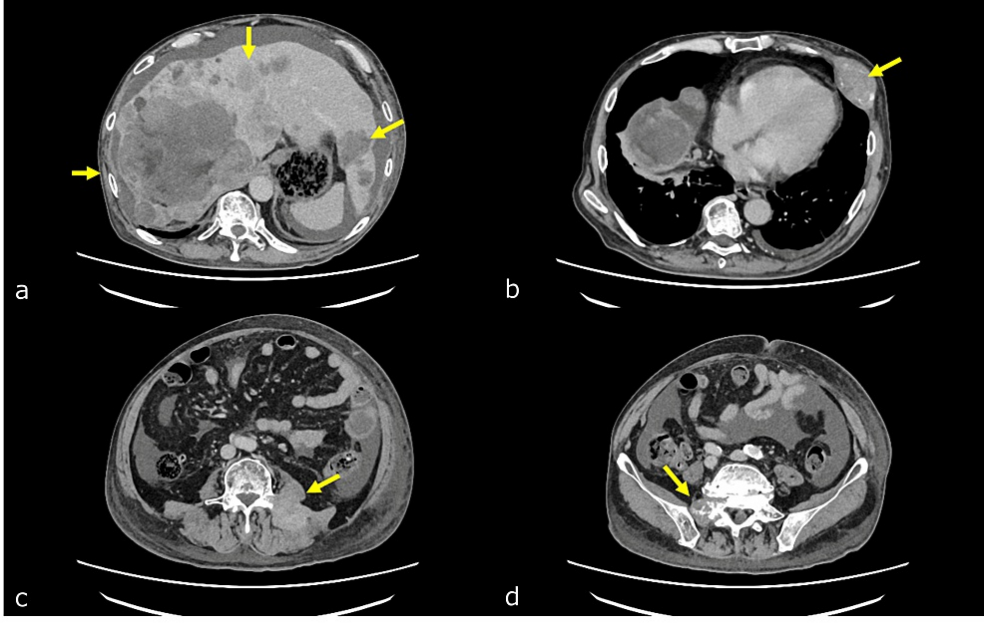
CT scan of the trunk A CT scan of the trunk, lumbar region, and pelvis revealed advanced liver metastases (a, indicated by yellow arrow) and metastatic tumors in the rib (b, indicated by yellow arrow), sacrum (c, indicated by yellow arrow), and sacrum (d, indicated by yellow arrow) (Figure 3).
